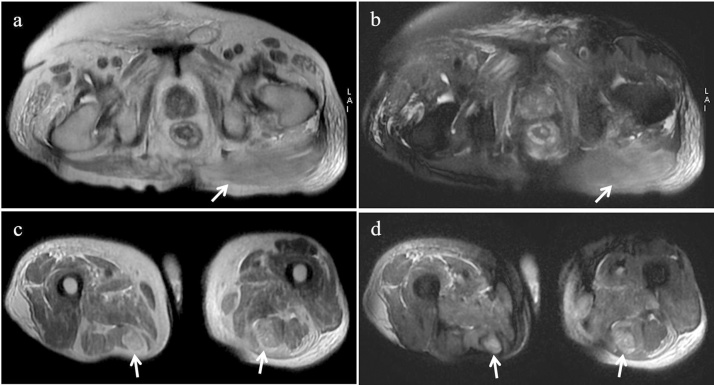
Muscle oedema on fluid-sensitive MRI sequences. Axial T2-weighted (a) and fat-suppressed T2-weighted (b) MR images show diffuse increased signal intensity of the left gluteus maximum muscle (white arrows). Axial T2-weighted (c) and fat-suppressed T2-weighted (d) MR images show atrophy and increased signal intensity of the right and left semitendinosus muscles (white arrows).
